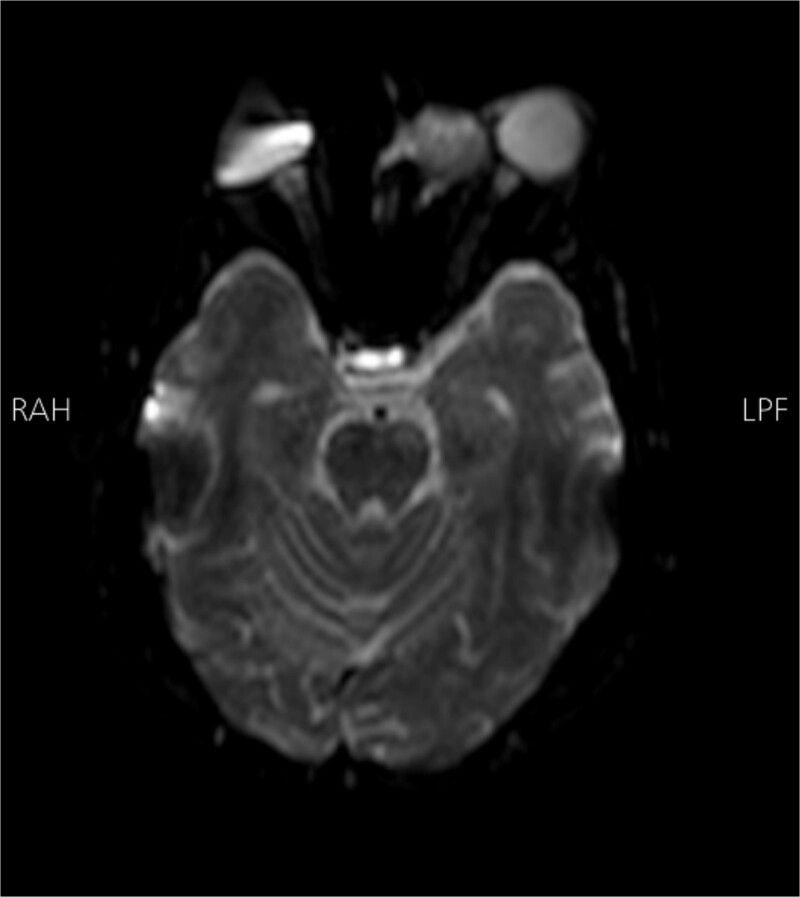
Brain MRI DWI sequence showing abnormal hyper-intense signal (bright) in the distribution of the left cavernous sinus. The left ethmoidal sinus synovial tumor is also noted.
